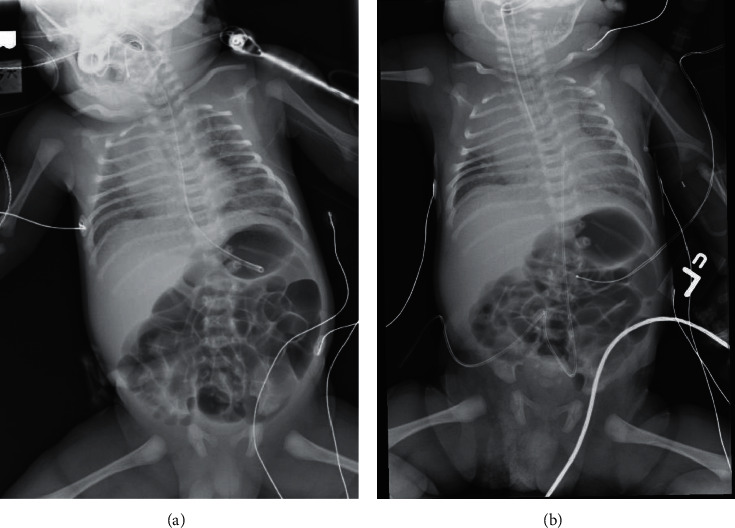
Radiograph of the chest and abdomen on admission. (a) The lungs are aerated bilaterally with LMA in place and show hazy parenchymal opacities bilaterally. There is a hemivertebra at T12 and 11 rib pairs on the right. (b) Similar radiograph with the esophageal tube in place in addition to removal of the nasogastric tube and placement of umbilical catheters.
